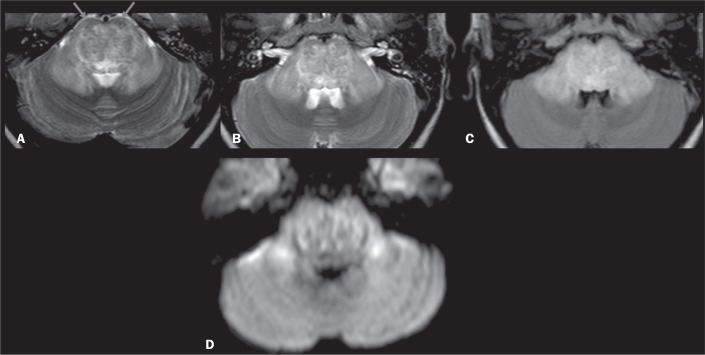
ODS. Axial T2WI (A,B) and axial FLAIR sequence (C), showing
